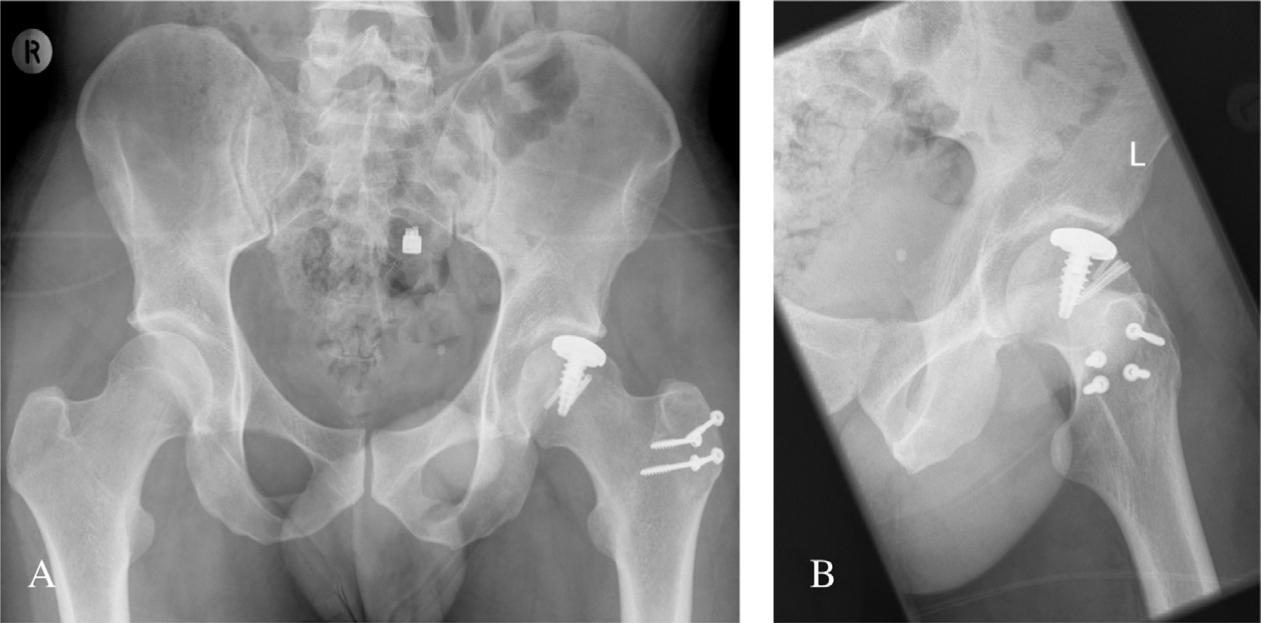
Patient 1: Final follow up X-ray at 7 years AP (A) and Lateral (B) View.
